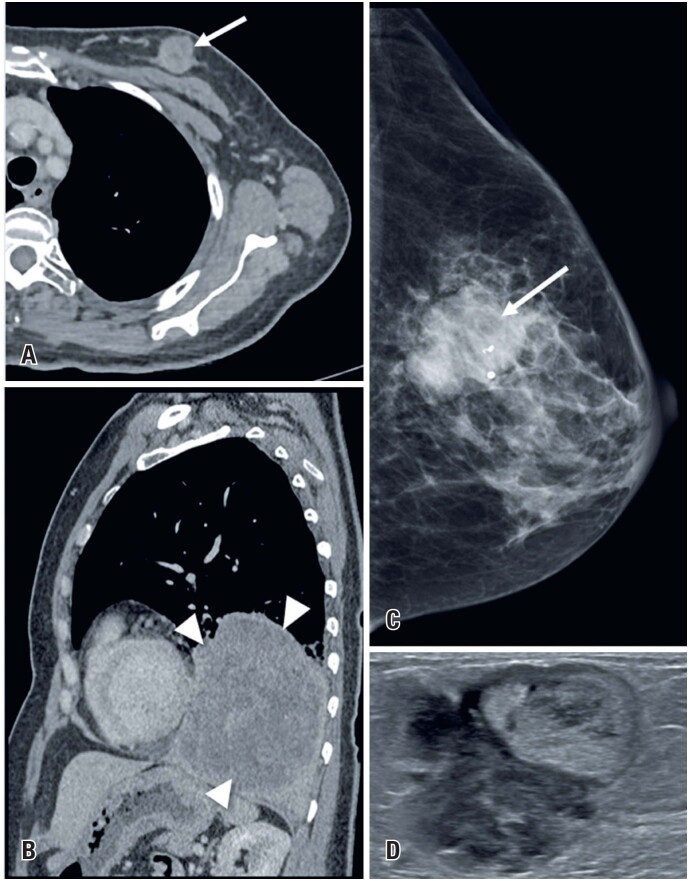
A 61-year-old woman undergoing treatment for small cell lung carcinoma had a follow-up chest CT. (A and B) In addition to the massive left lung lesion (arrowheads), a round mass was visualized in the left breast (arrow). (C) Mammography, showed an irregular obscured mass (arrow) classified as BI-RADS 4. (D) On ultrasound, it appeared as a solid-cystic lesion. A biopsy confirmed metastatic lung carcinoma
